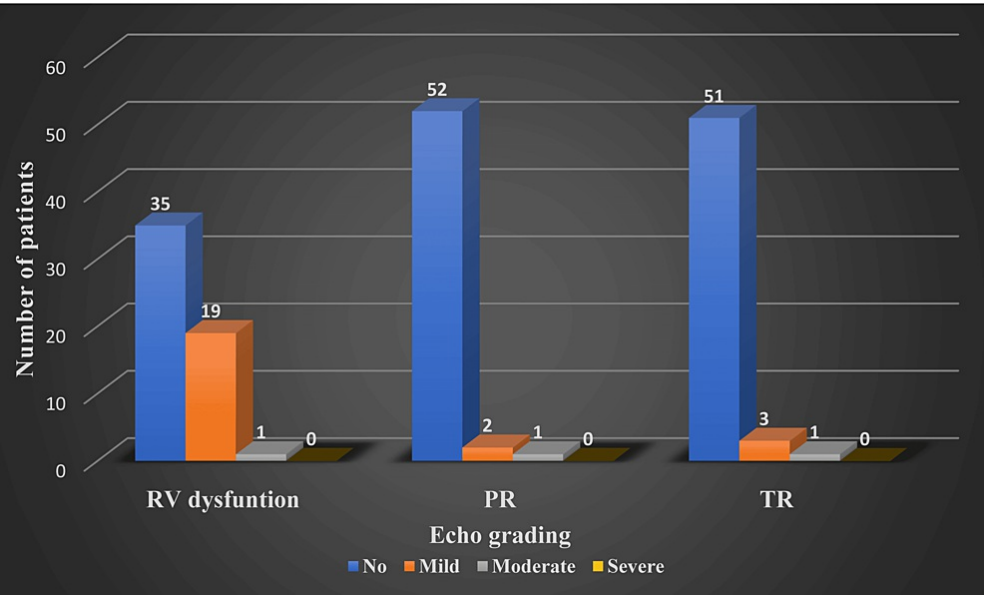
Follow-up echocardiography reported complications. RV = right ventricular; PR = pulmonary valve regurgitation; TR = tricuspid valve regurgitation
